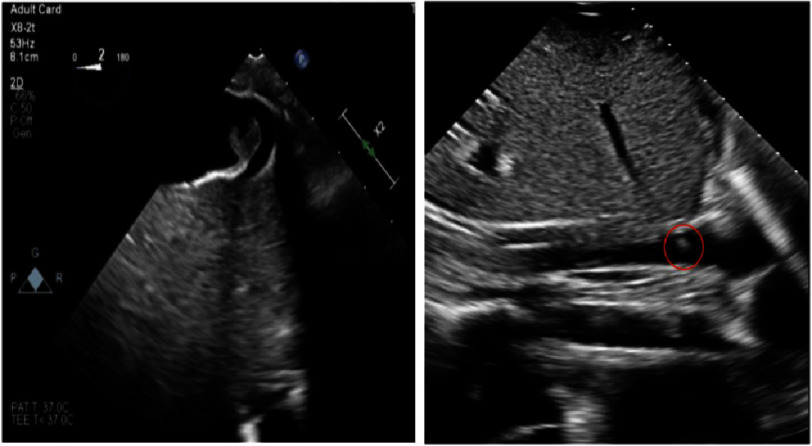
(a, b) Transthoracic echocardiography showing a mobile mass measuring 10 mm × 7 mm just distal to the hepatic vein.
